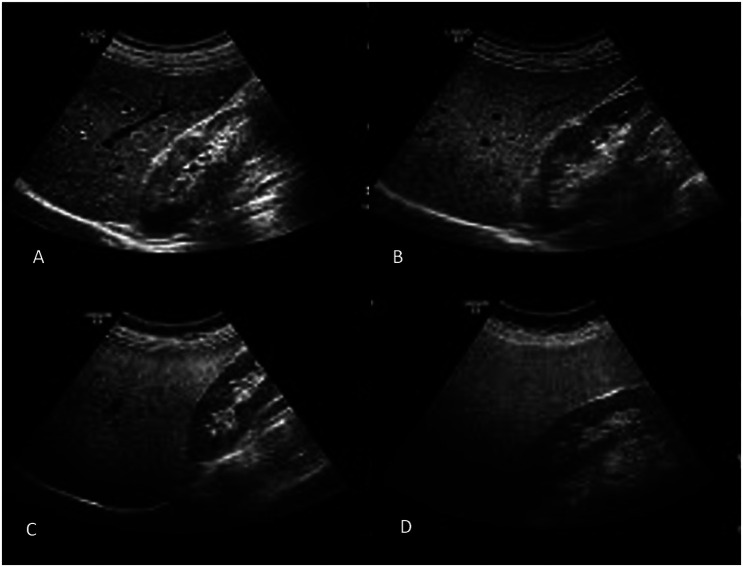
Ultrasonographic grading of hepatosteatosis.
